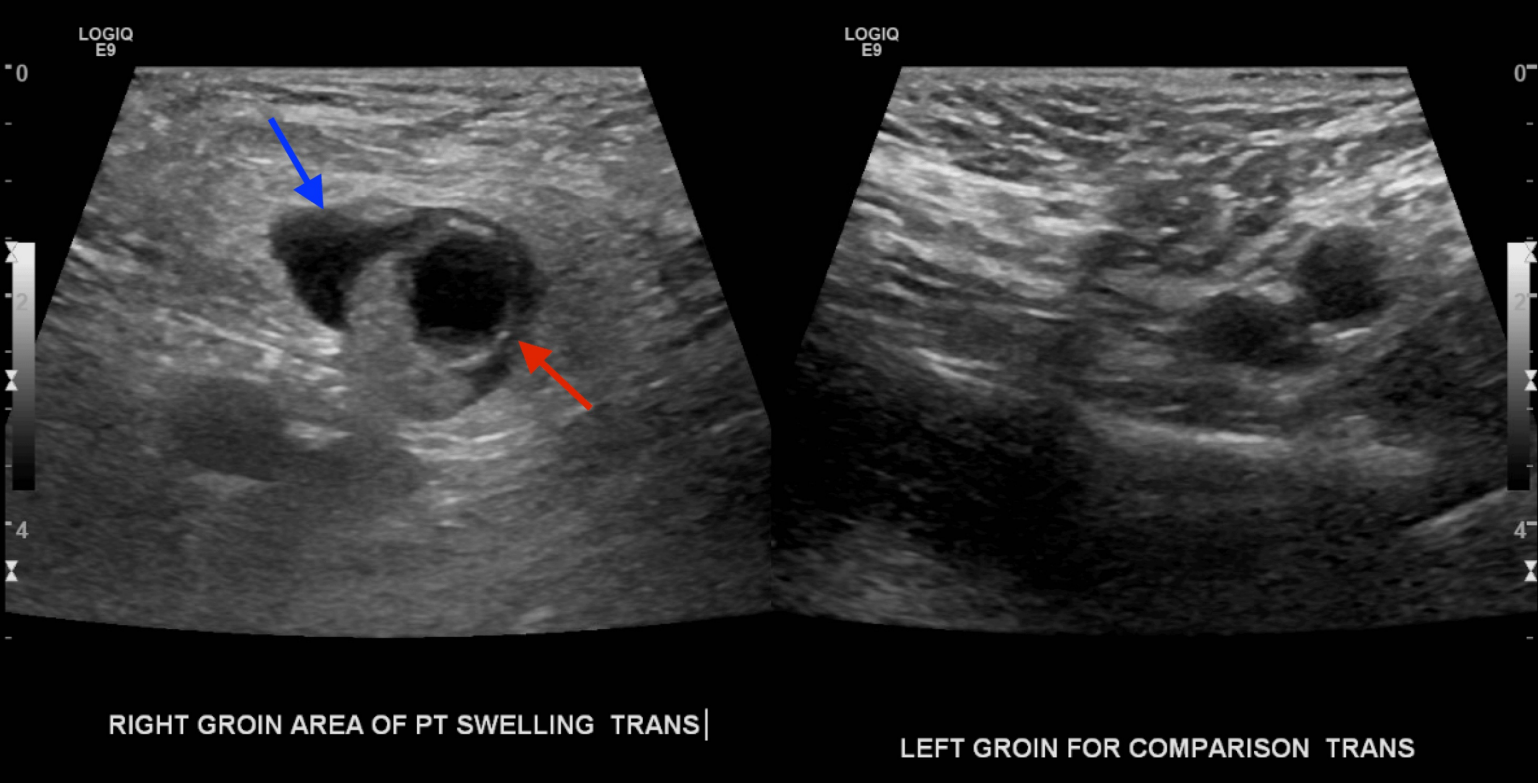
Ultrasound of the right and left groin Comparison of the transverse view of the right and left groin regions with the femoral hernia (blue solid arrow) and a cystic structure within (red solid arrow) present in the right groin.
